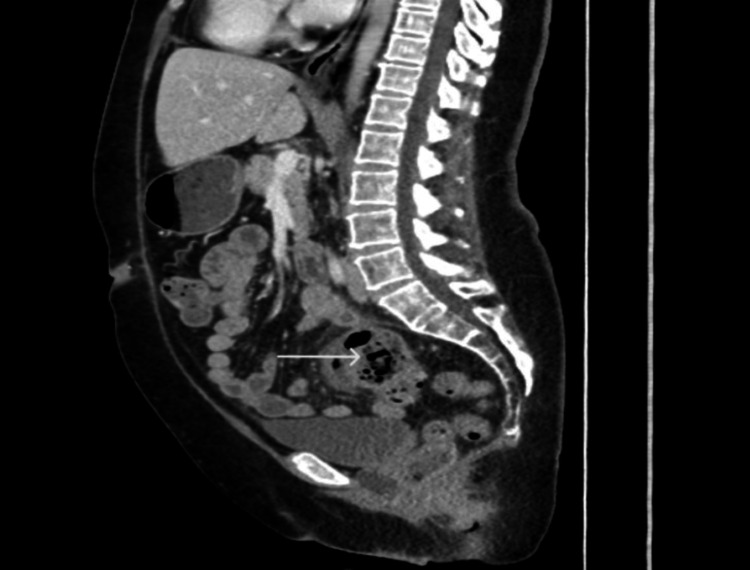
CT abdomen and pelvis sagittal view CT of the abdomen and pelvis (sagittal) demonstrating sigmoid GCD (arrow) with associated surrounding inflammation.
